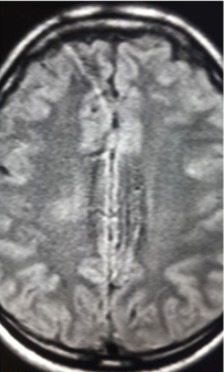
Computed tomography image of the patient brain
